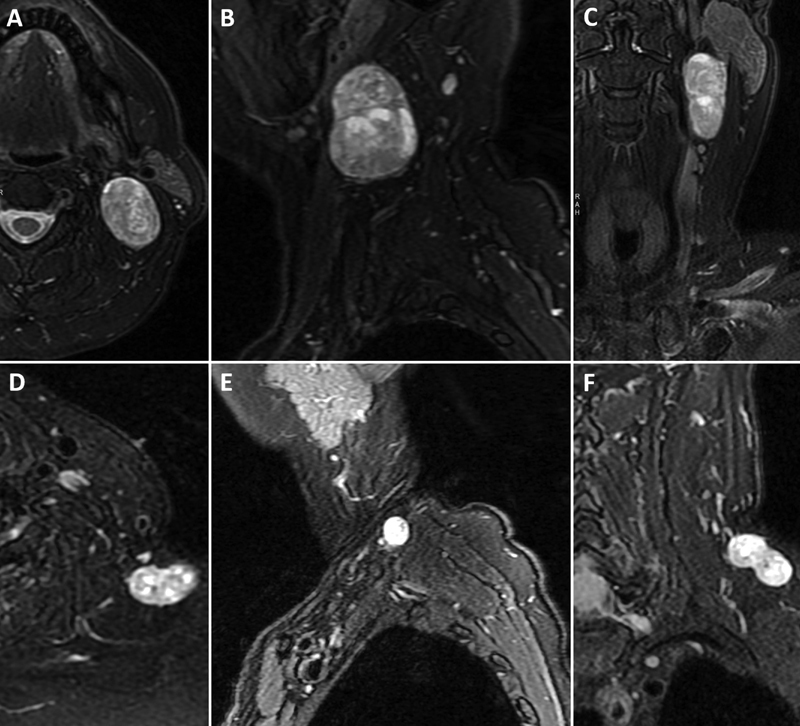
Magnetic resonance imaging (MRI) neurogram demonstrated a larger 4.6 × 3.2 × 2.5 cm mass deep to the left sternocleidomastoid muscle just below the angle of the mandible
